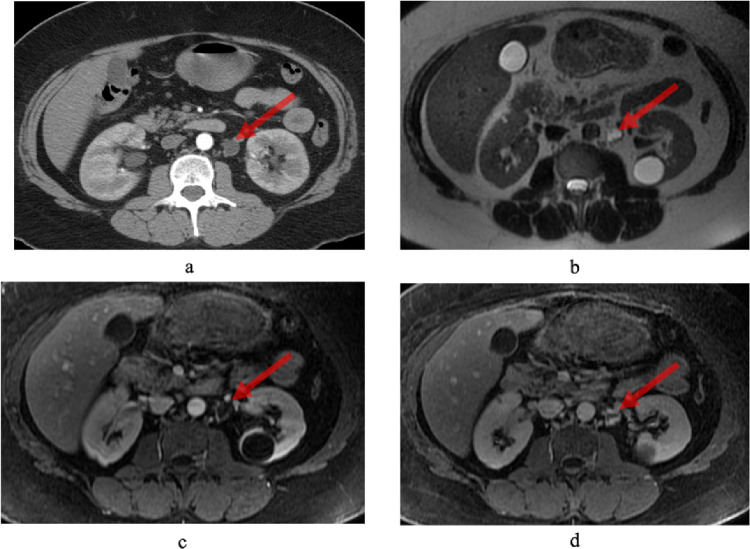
Retroperitoneal Hemangioma. An axial contrast-enhanced CT image (a) shows a hypodense left paraaortic lesion with incomplete peripheral enhancement (red arrow). The lesion is hyperintense on an axial T2-weighted image (b) and demonstrates incomplete peripheral nodular enhancement with progressive centripetal fill-in on axial fat suppressed contrast-enhanced T1-weighted images (c, d), features compatible with a retroperitoneal hemangioma
